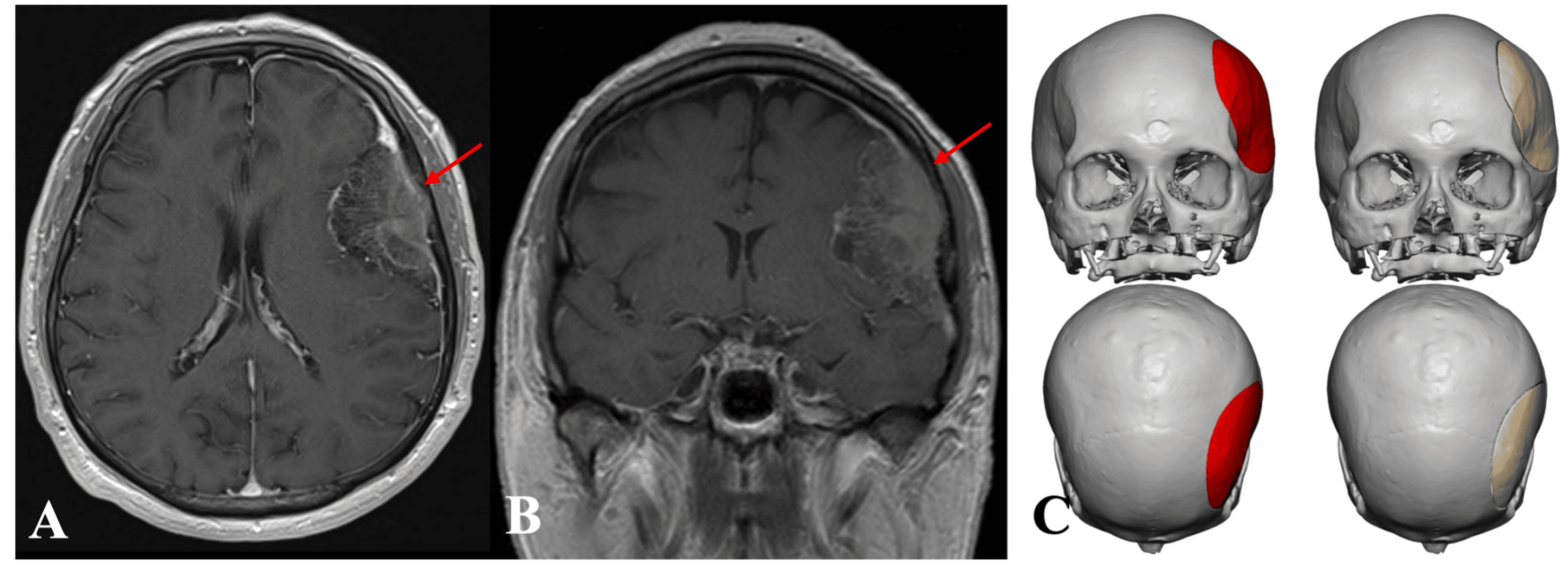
(A) Axial and (B) coronal preoperative images of MRI brain (T1-weighted with contrast) showing left convexity tumor (red arrow). (C) Preoperative PEEK implant design images MRI: magnetic resonance imaging; PEEK: polyetheretherketone
